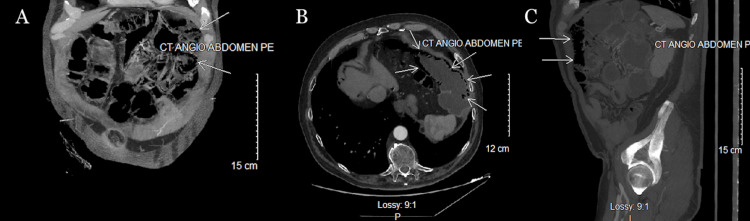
CTA scan showing increased bowel pneumatosis and dilation. A) Coronal view. B) Axial view. C) Sagittal view. This is an original CTA scan of the patient detailed by this report. Pathology indicated by white arrows. CTA: computed tomography angiography.
